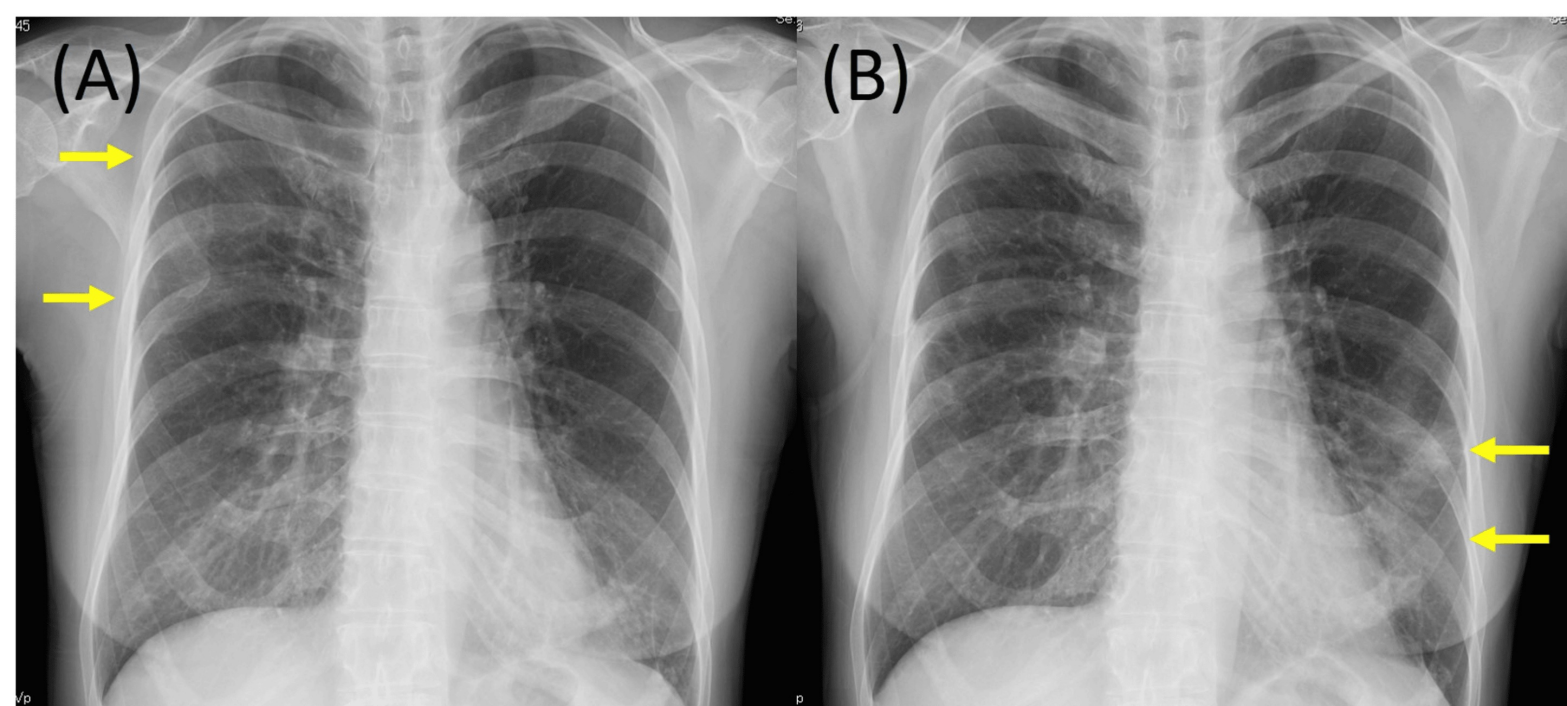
Chest X-ray in case 1 (A) Chest X-ray 1 month before the first consultation showing ground-glass opacities (GGOs) in the right upper lung. (B) Repeat chest X-ray at the first consultation showing improved previous opacities but a new GGO in the left lung.
